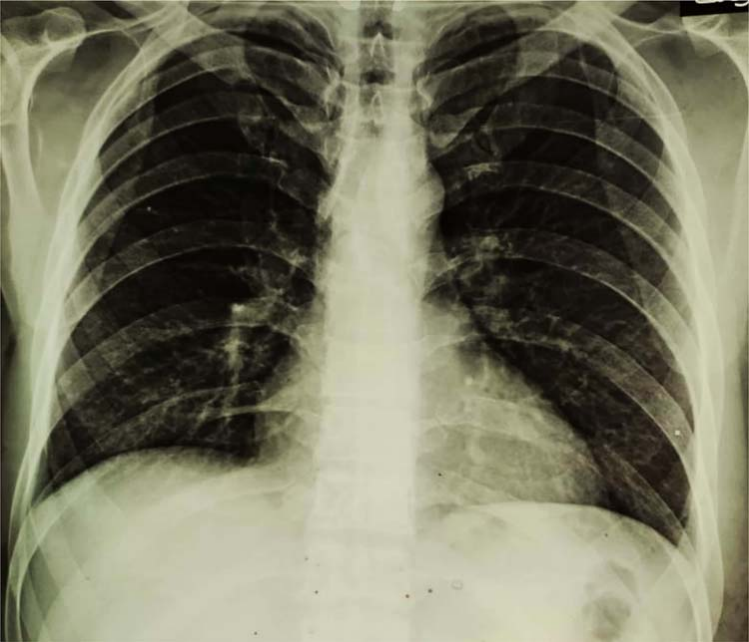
Chest X-ray PA view labelled as normal.
